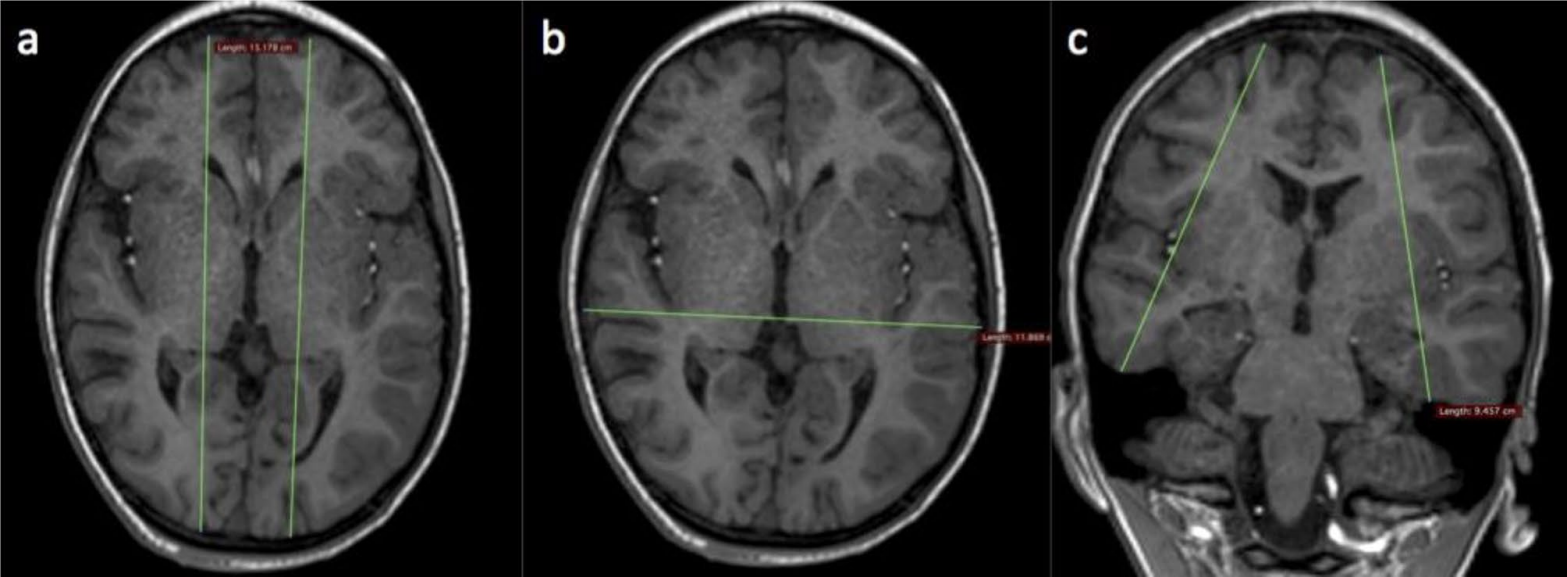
Linear measures on T1W MRI images. Fronto-occipital measures in the axial plane (a). Bi-temporal or bi-parietal diameter in the axial plane (b). Hemispheric height in the coronal plane (c)
